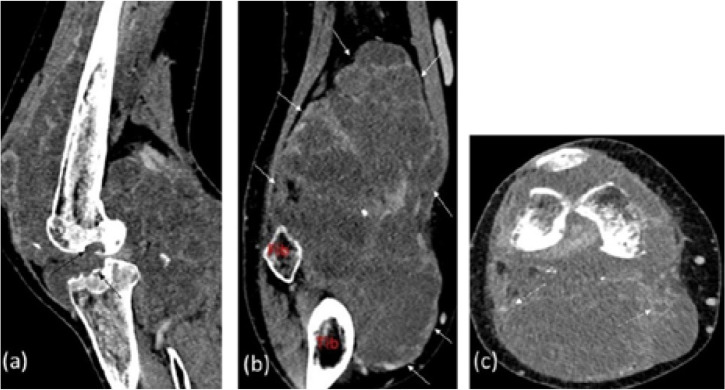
RIGHT Knee CT with post contrast. Sagittal (a), coronal (b), axial (c). Erosive changes and bony destruction (black arrows). There is a large septate lesion with intra and extra-articular components (within white arrows on b) that demonstrates enhancement (dashed white arrows) on the postcontrast imaging predominantly along the septations.
